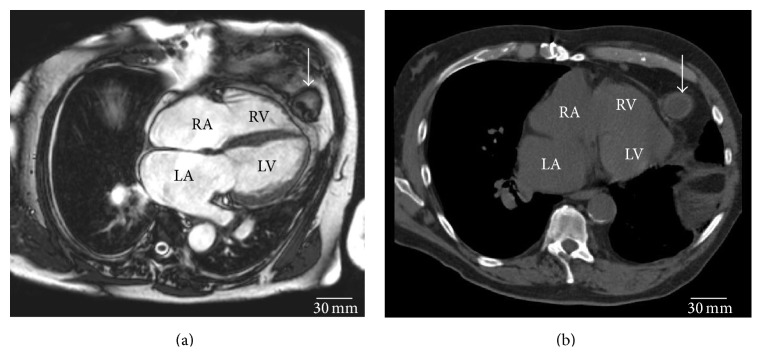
Epicardial foreign body (arrow) with a diameter of 30 mm in (a) cardiac magnetic resonance imaging (MRI) and (b) thoracic computed tomography (CT): diffuse thickening of the pericardium surrounding the foreign body with pericardial effusion is shown. RA denotes right atrium, LA left atrium, RV right ventricle, and LV left ventricle.
